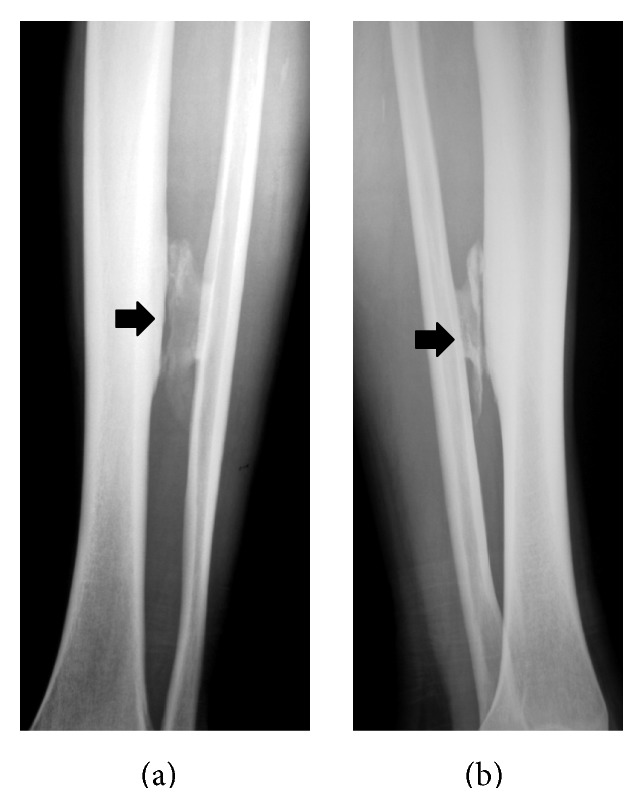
Anteroposterior (a) and lateral-oblique (b) radiographs of the left leg showing ossification in the middle third of the leg (*arrows*), possibly located in the interosseous membrane.
